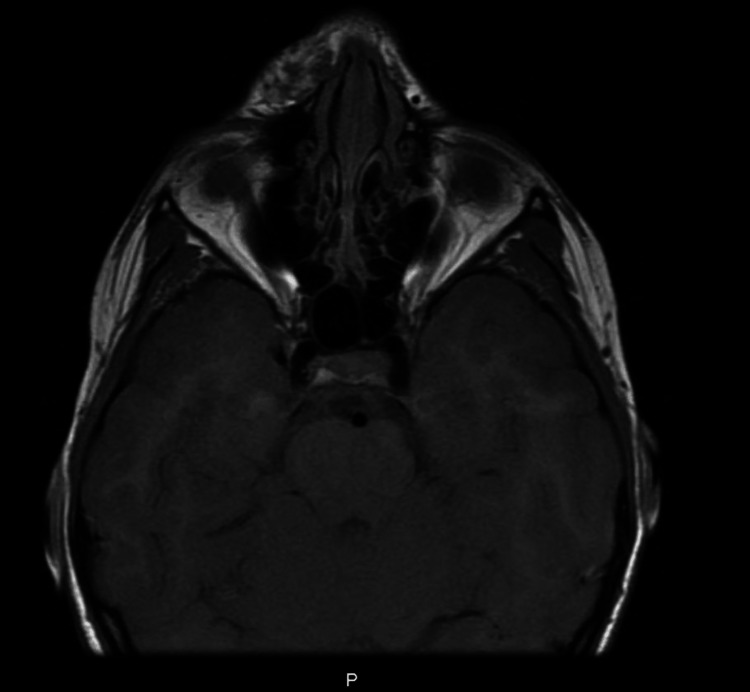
MRI scan of the facial soft tissue with contrast (white arrow) showing vascular lesion centered in the right nasal ala and nasolabial fold
